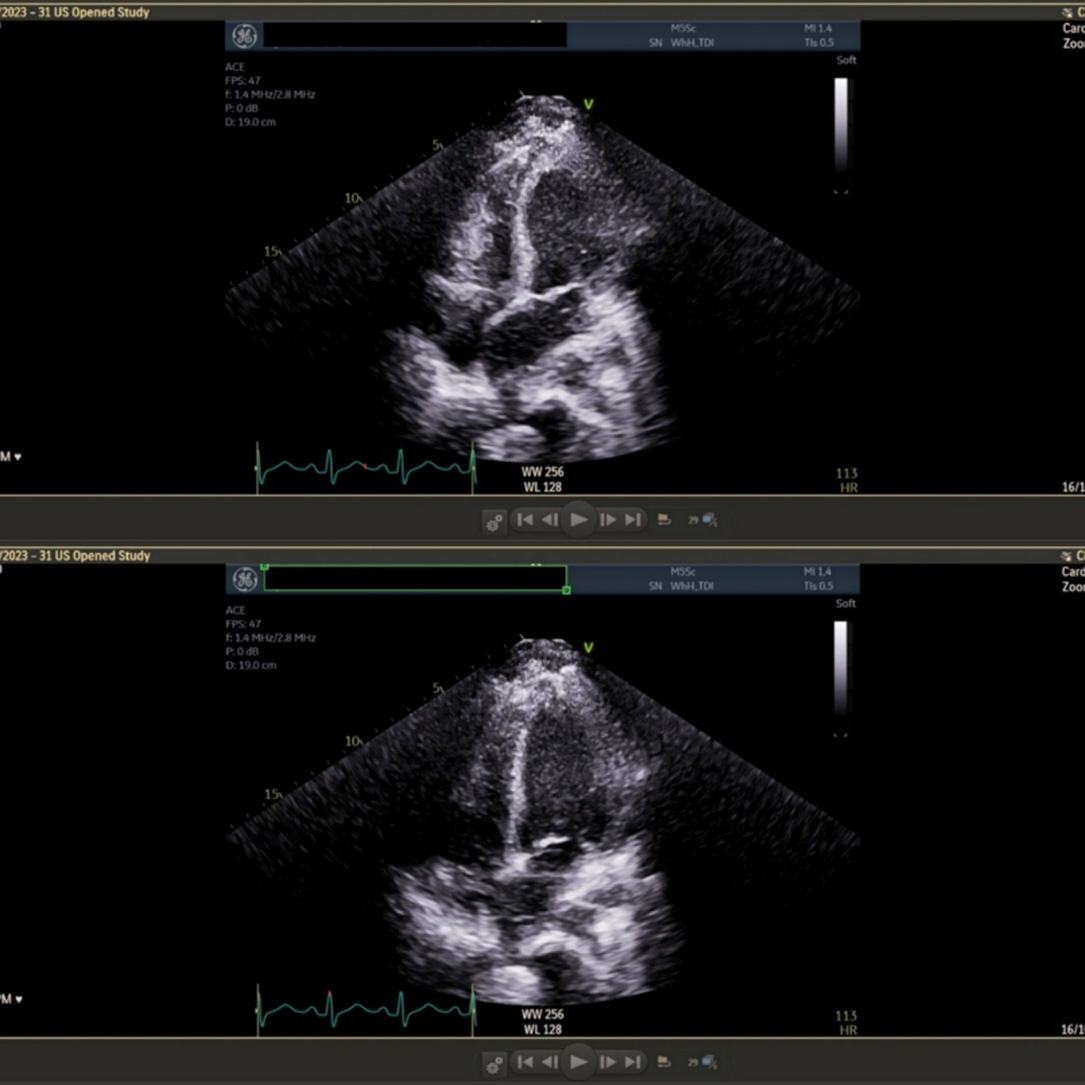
Transthoracic echocardiogram demonstrating mid‐segment hypokinesia of the ventricular septum during systole (top). An image of diastole (bottom) is included for comparison. A video recording of the investigation can be found in the Supporting information.
